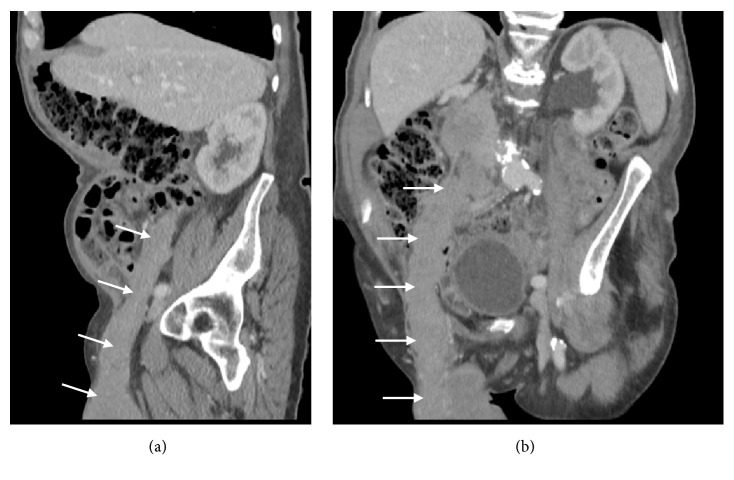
(a and b) Sagittal and coronal reformatted CT images of the abdomen and pelvis with contrast demonstrating cranial extension of the tumour from the scrotum, along the spermatic cord in the inguinal canal and into the retroperitoneum along the right gonadal vein, to the level of its insertion into the inferior vena cava. Incidental note made of a left extrarenal pelvis.
